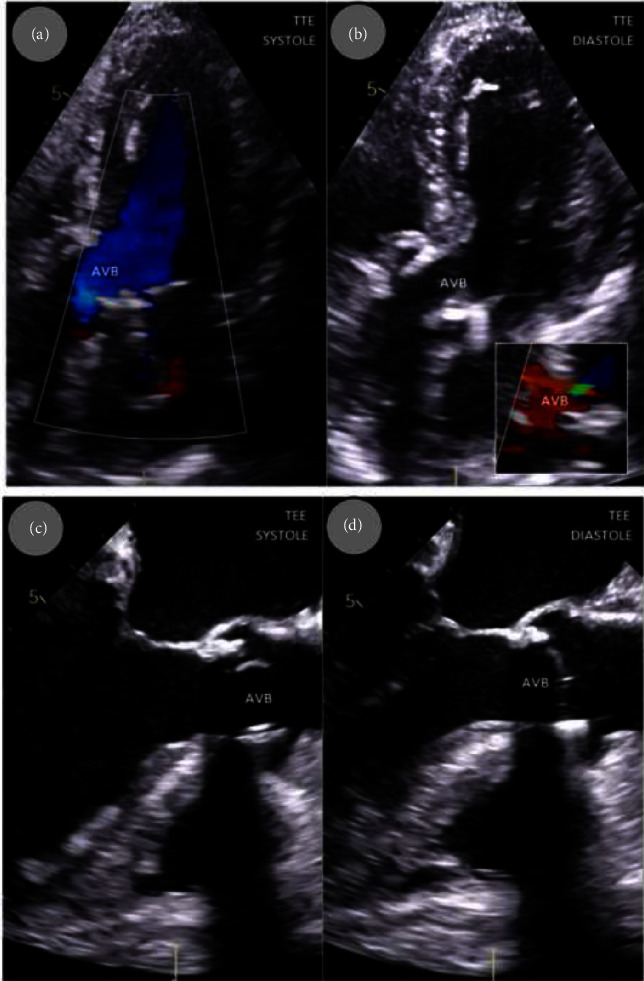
Echocardiography after aortic valve bioprosthesis implantation: normal color flow during systole (a), trace aortic regurgitation seen in diastole—zoom in lower/right site (b); normal aortic valve bioprosthesis (AVB) without vegetation seen in transesophageal echocardiography in systole (c); and diastole (d). TEE, transesophageal echocardiography.
